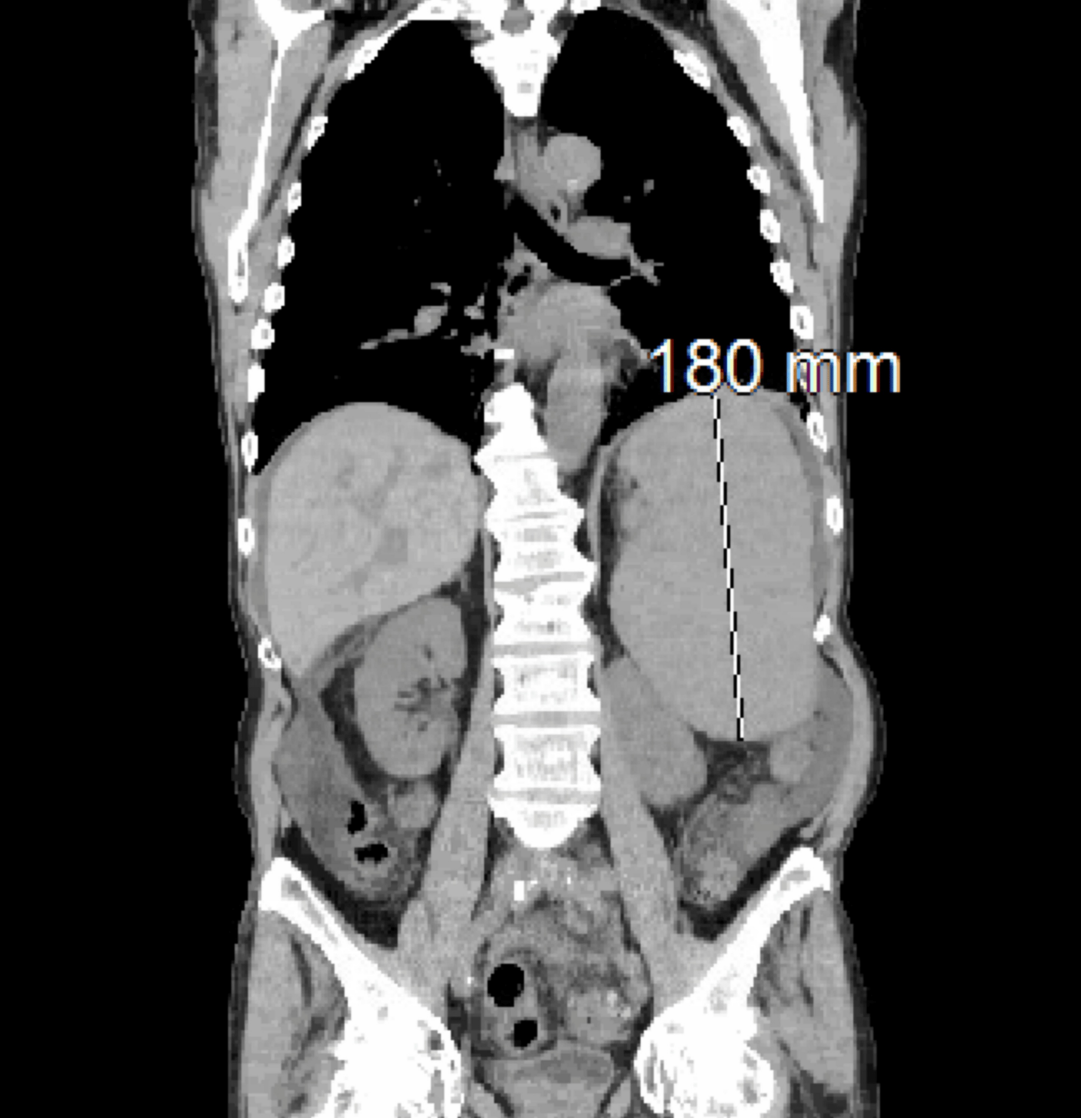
Abdominal CT, June 12, 2014. The abdominal CT reveals splenomegaly with a craniocaudal length of 18 cm, supporting a diagnosis of splenic marginal zone lymphoma.
